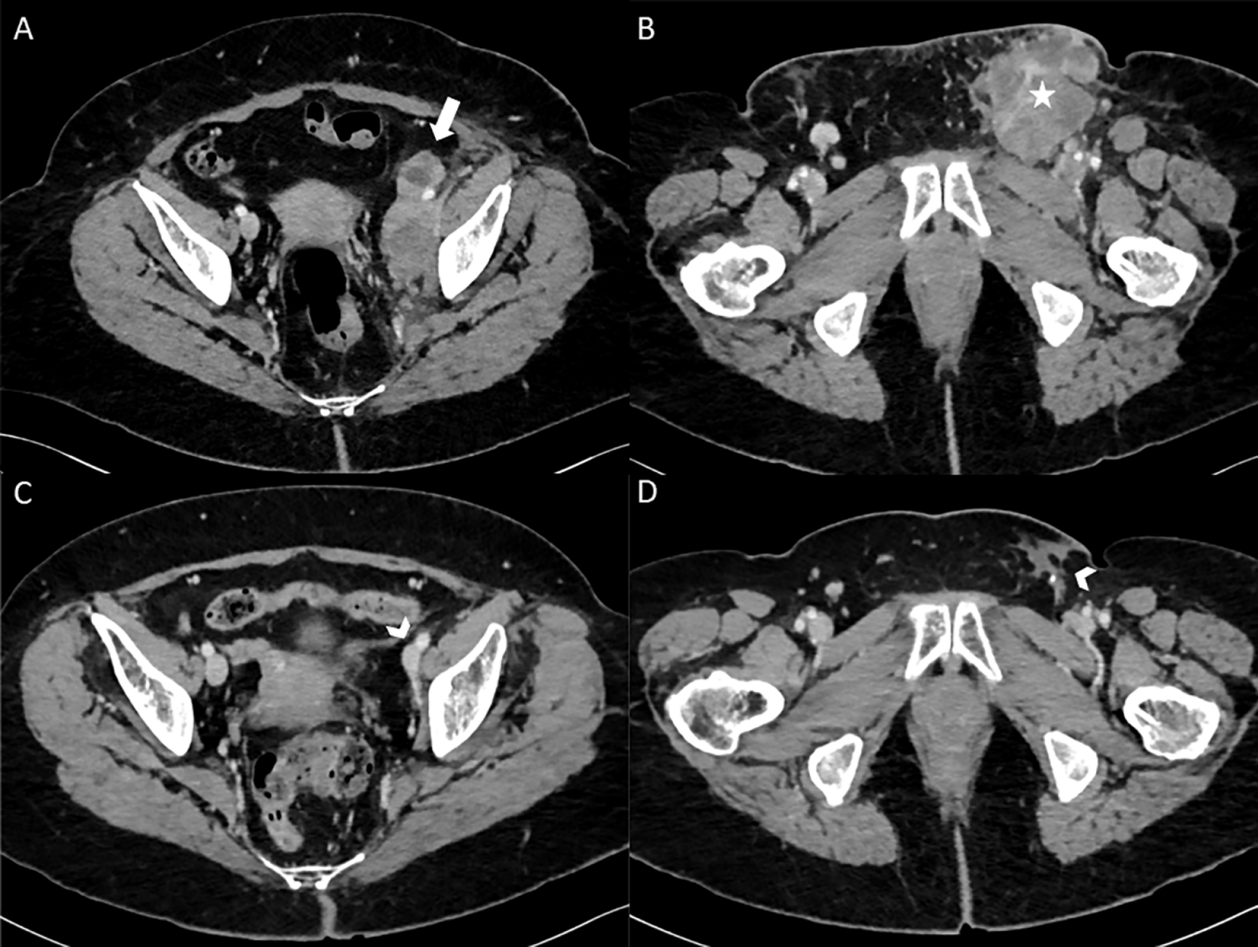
Enhanced CT scanner images (axial view) at baseline in February 2020 (A, B) showing left external and internal iliac lymphadenopathy (thick white arrow) and left inguinal lymphadenopathy (white star). After 1 Ipilimumab/Nivolumab infusion with 3.5 years of follow-up in August 2023 (C, D), enhanced CT scanner (axial view) only report lymph nodes remodeling signs (arrowheads) without any sign of active tumors.
